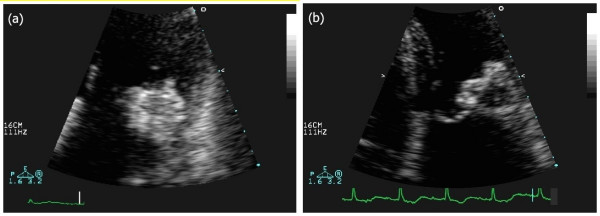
Two-dimensional echocardiogram, apical four-chamber view (detail). (a) Pre-operative: dyshomogeneous echodensity of the mass is evident. (b) Postoperative: the mass has central echolucency surrounded by a hyperechogenic region.
